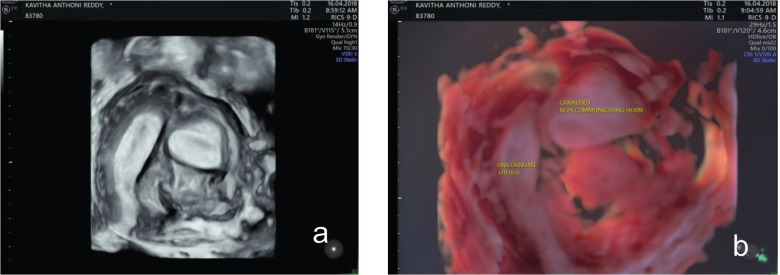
(a) A small communicating uterine cavity on the right side and a blind uterine cavity on the left side in a 3D-rendered vaginal ultrasonic view (b) 3D live reconstruction.
